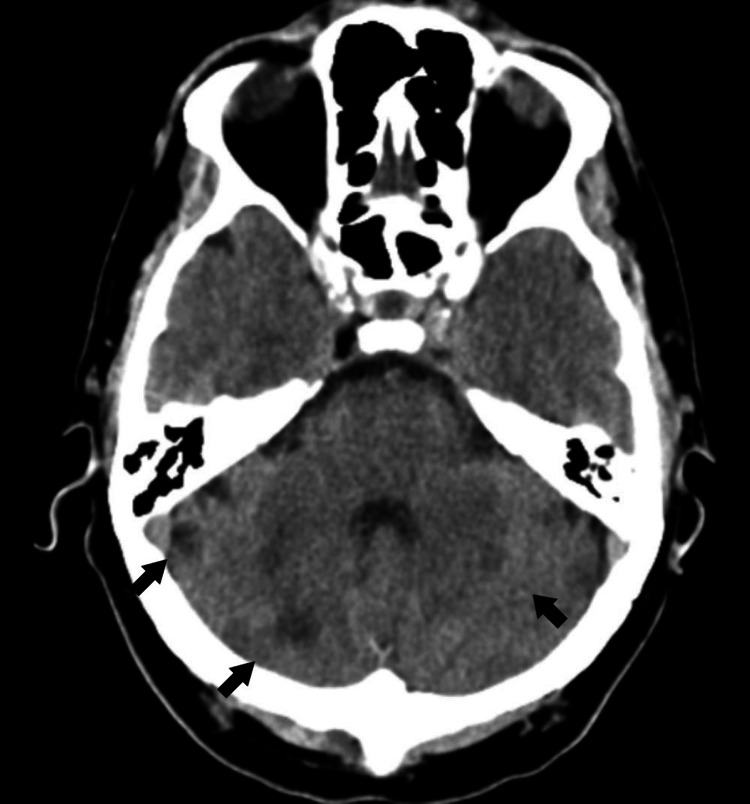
Admission brain-CT showing multiple and bilateral cerebellar ischemic lesions (arrows). CT, computed tomography.
